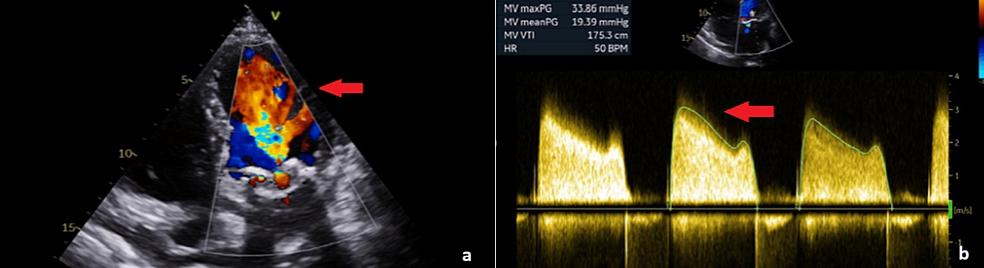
a. Color Doppler in the echocardiogram with accelerated velocities through the bioprosthetic valve (red arrow); b. Severe stenosis of the bioprosthetic valve with a meanPG 19.39 mmHg (red arrow).
